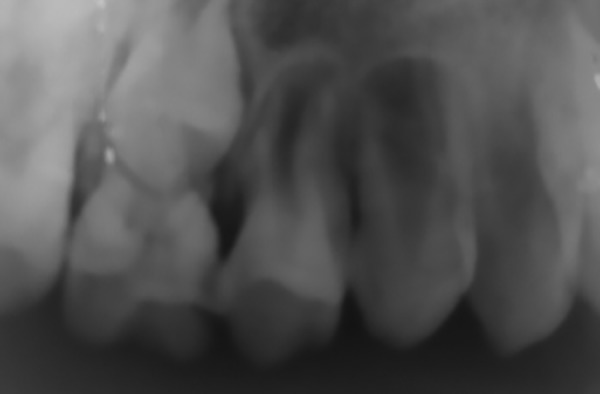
Postoperative 20 months follow-up radiograph
